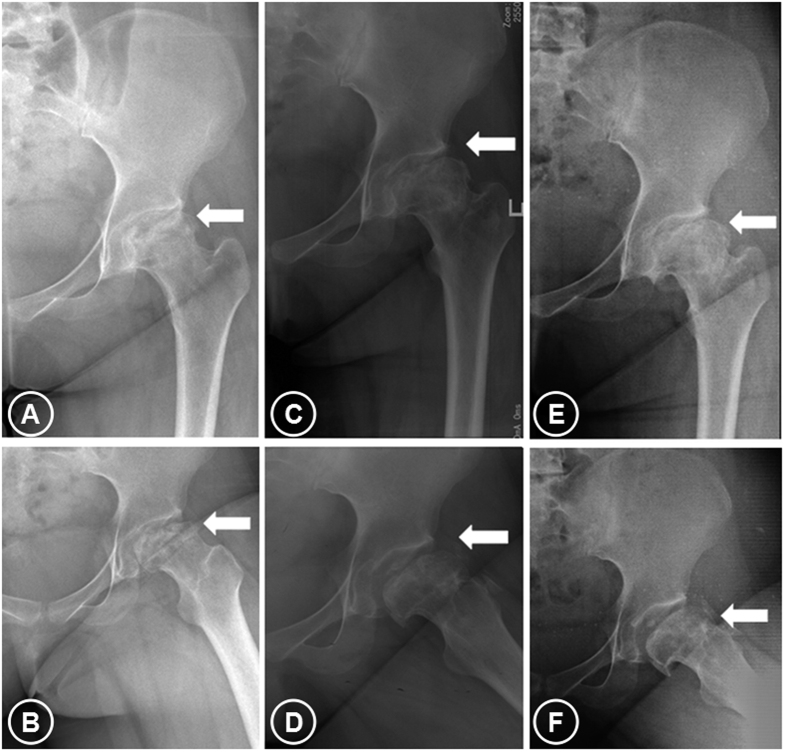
The second patient, 15-year-old girl: the osteonecrosis of the femoral head in her left hip 6 months after internal fixation removal following femoral neck fracture (ARCO Stage IIIc, CJFH Type L3). Preoperative x-ray (A, anteroposterior view; B, frog lateral view) showing already collapse of the necrotic lesion of the left femoral head after fracture; x-ray at 3 years after the combined treatment (C, anteroposterior view; D, frog lateral view), showing femoral head flattening and no further collapse, yet with stable reshaping; x-ray after 6 years (E, anteroposterior view; F, frog lateral view), showing no further collapse and the shape of the femoral head conserved (arrows).
